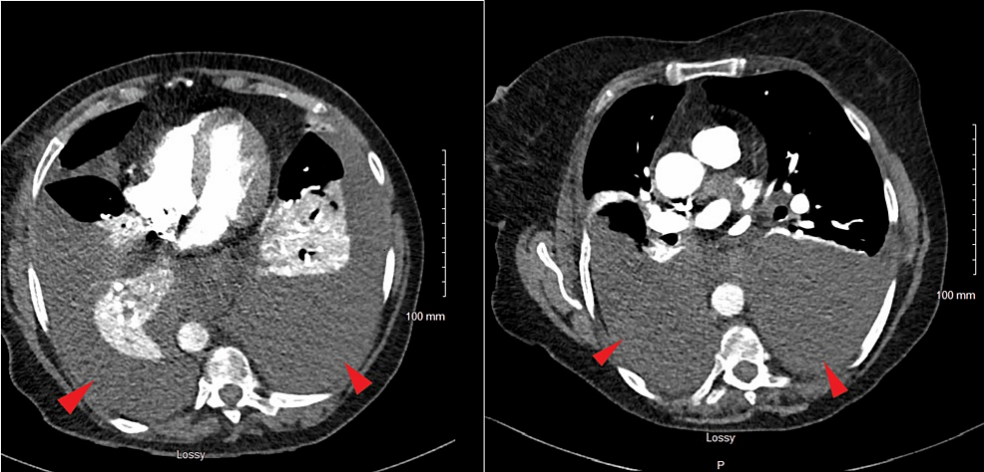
CTPA showing bilateral large pleural effusions with extensive bilateral lower lobe atelectasis; the aerated lungs are clear. CTPA: computed tomography pulmonary angiogram
